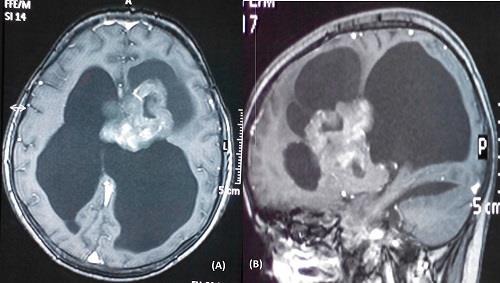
figures, contrast T1-weighted axial (A) and sagittal (B) magnetic resonance images of a child showing an intraventricular contrast enhancing lesion with hydrocephalus, features compati-ble with of choroid plexus papilloma.
